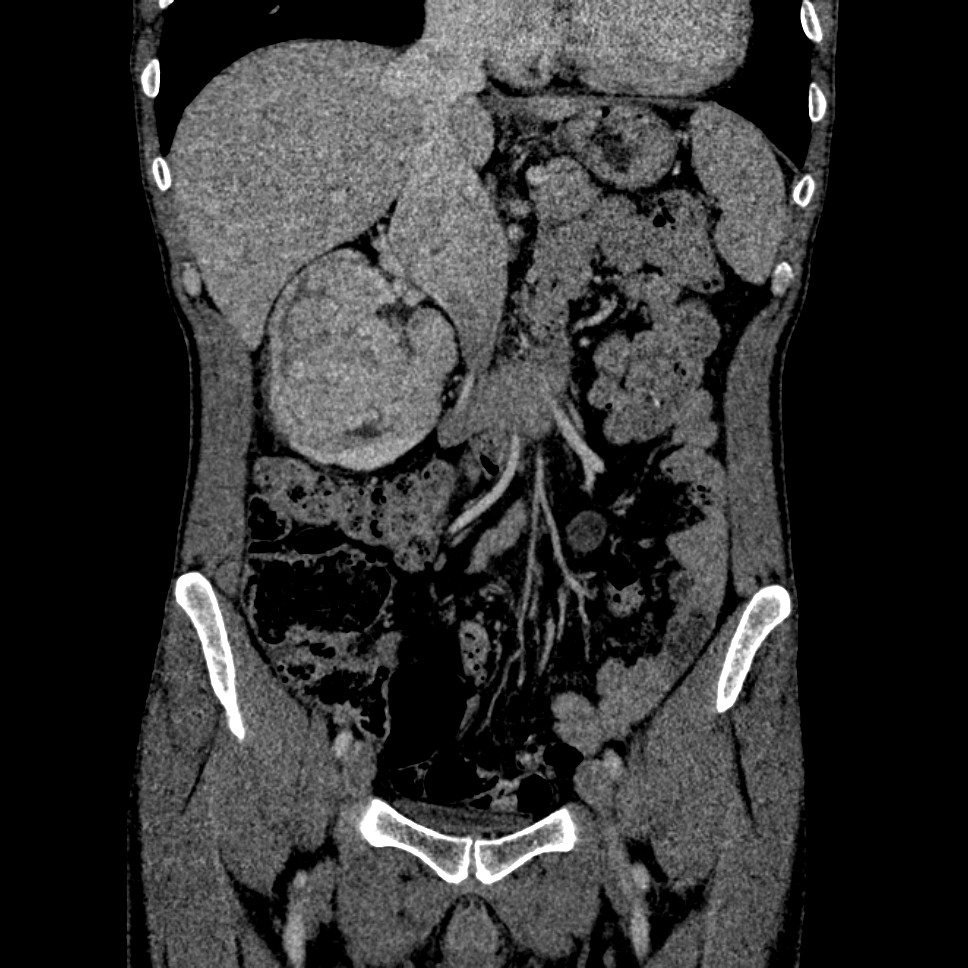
CT reconstruction of the abdomen showing the size of the renal mass and the thrombus.
